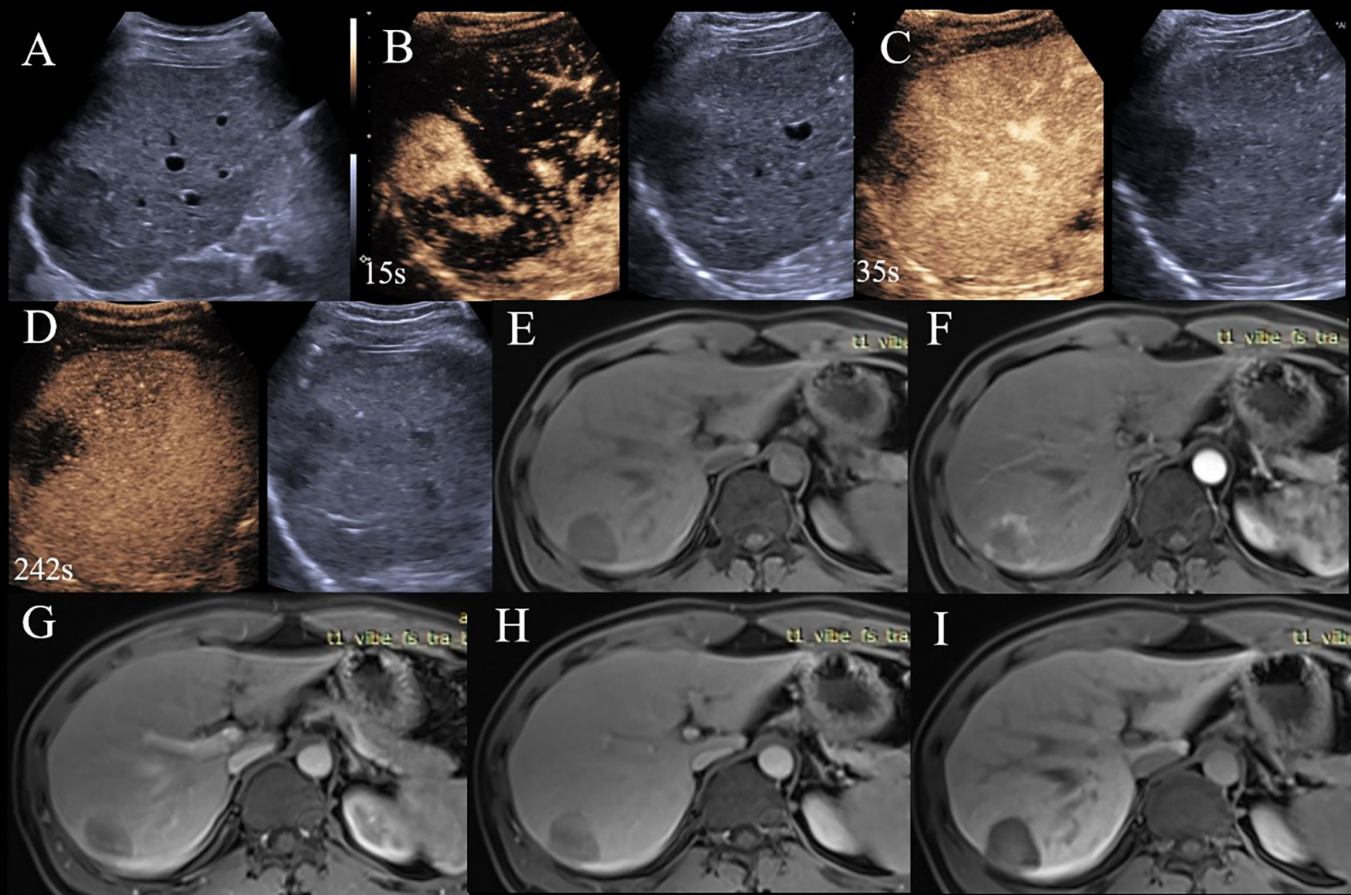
A 36-year-old man with HBV related liver cirrhosis. Serologic data indicated AFP of 167 ng/ml and CA19-9 of 13.5 ng/ml. (A) A hypo echoic lesion located under the liver capsule with the size of 4.1×3.8cm. (B) The lesion displayed APHE on CEUE; (C) Early washout was observed at 35s after injection of contrast agent; (D) Washout was observed on delay phase, the lesion was categorized as CEUS LR-M. (E) The lesion displayed hypointensity on EOB-MRI; (F) Rim-APHE was observed on EOB-MRI; (G, H) Delayed enhancement was also observed on portal and transitional phases; (I) The lesion showed hypointensity on hepatobiliary phase, the lesion was EOB MRI LR-M. The final diagnosis was cHCC-CCA.
